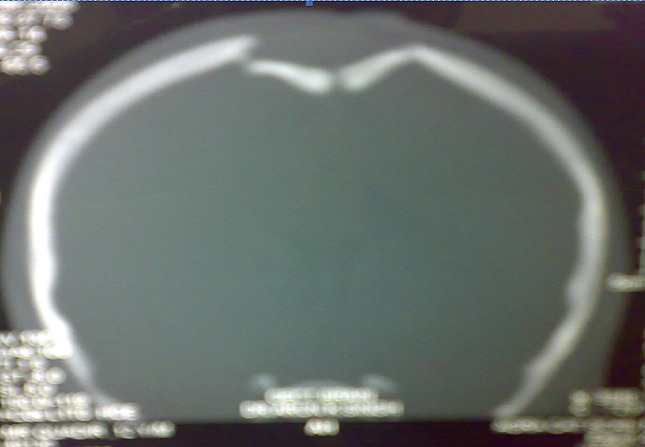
CT scan of the head showing bilateral depressed parietal bone fracture
